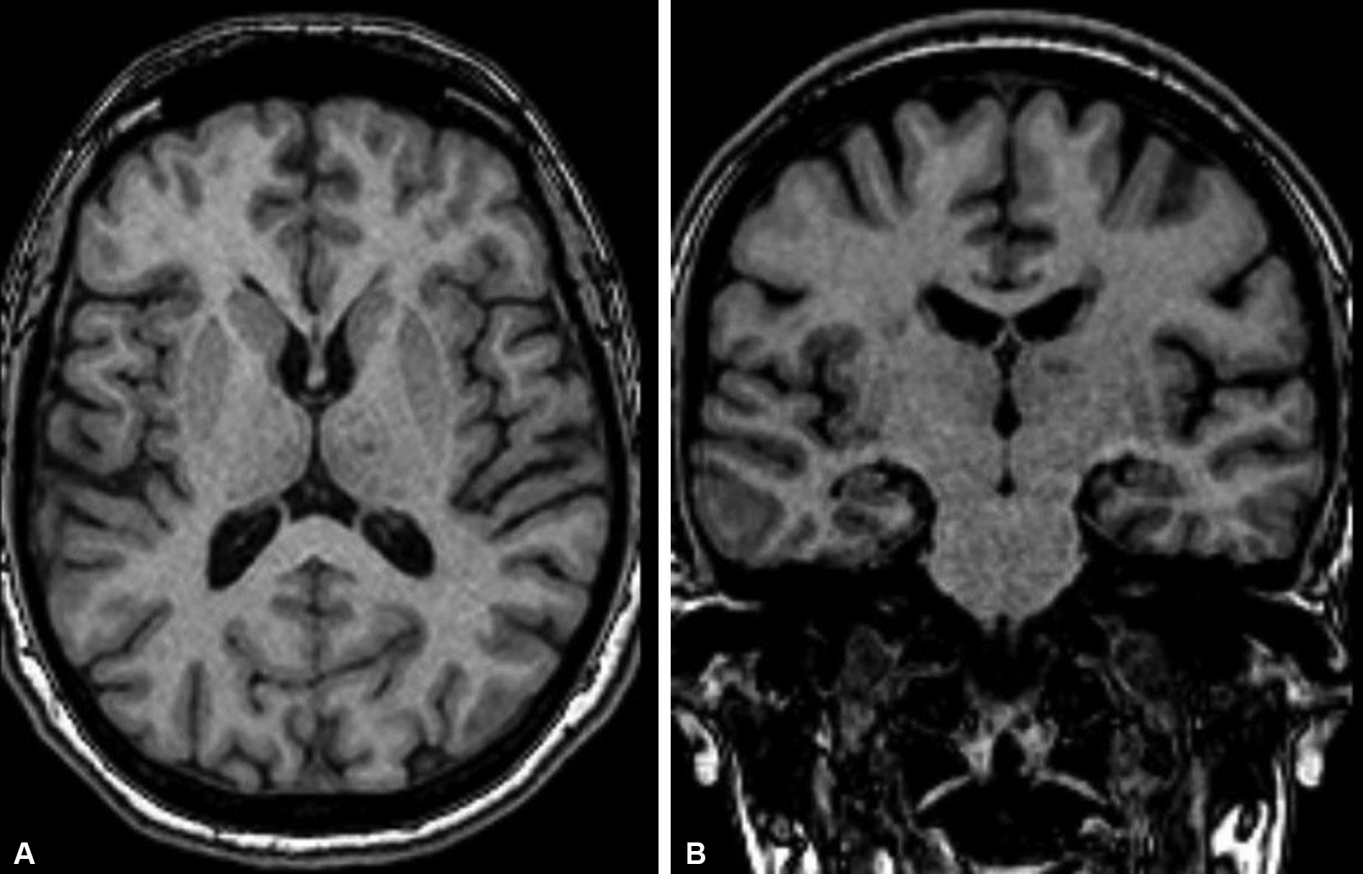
A. High resolution 3D-T1 MR images in axial plane; B. High resolution 3D-T1 MR images in coronal planes.
